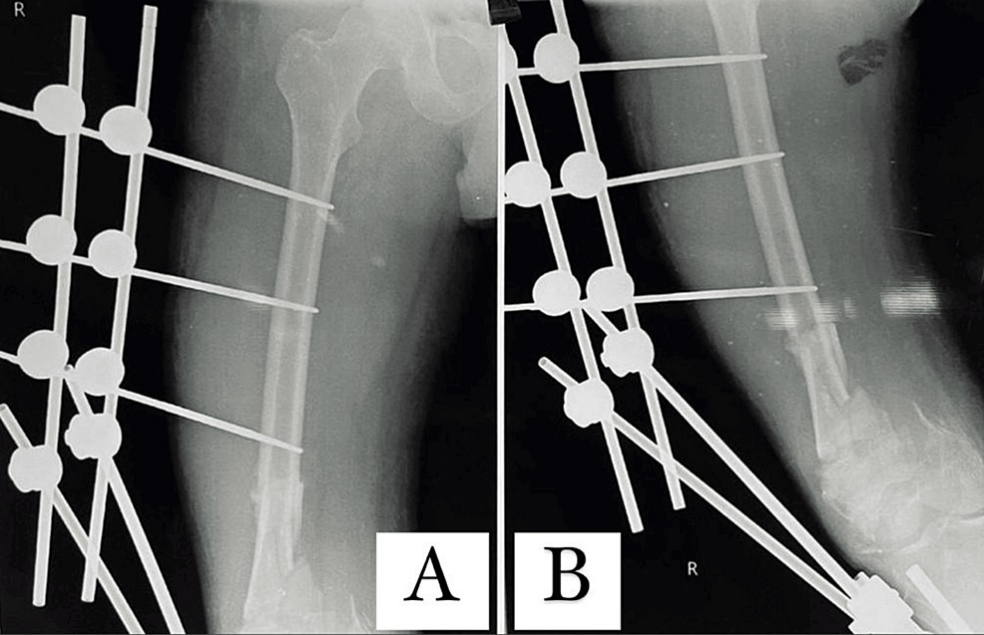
X-ray of non-union of shaft of femur is evident (A) Anterior view; (B) lateral view In anterior view and lateral view of X-ray, external fixator is seen attached to shaft of femur
